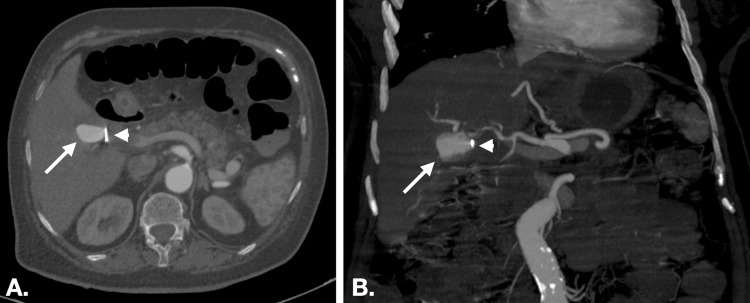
CT image showing the hepatic pseudoaneurysm adjacent to the right hepatic artery, with the larger arrow pointing to the pseudoaneurysm and the smaller arrow indicating the vascular metallic clip (from previous laparoscopic cholecystectomy) positioned tangentially to the PA. (A) Axial view. (B) Coronal view. PA: Pseudoaneurysm
